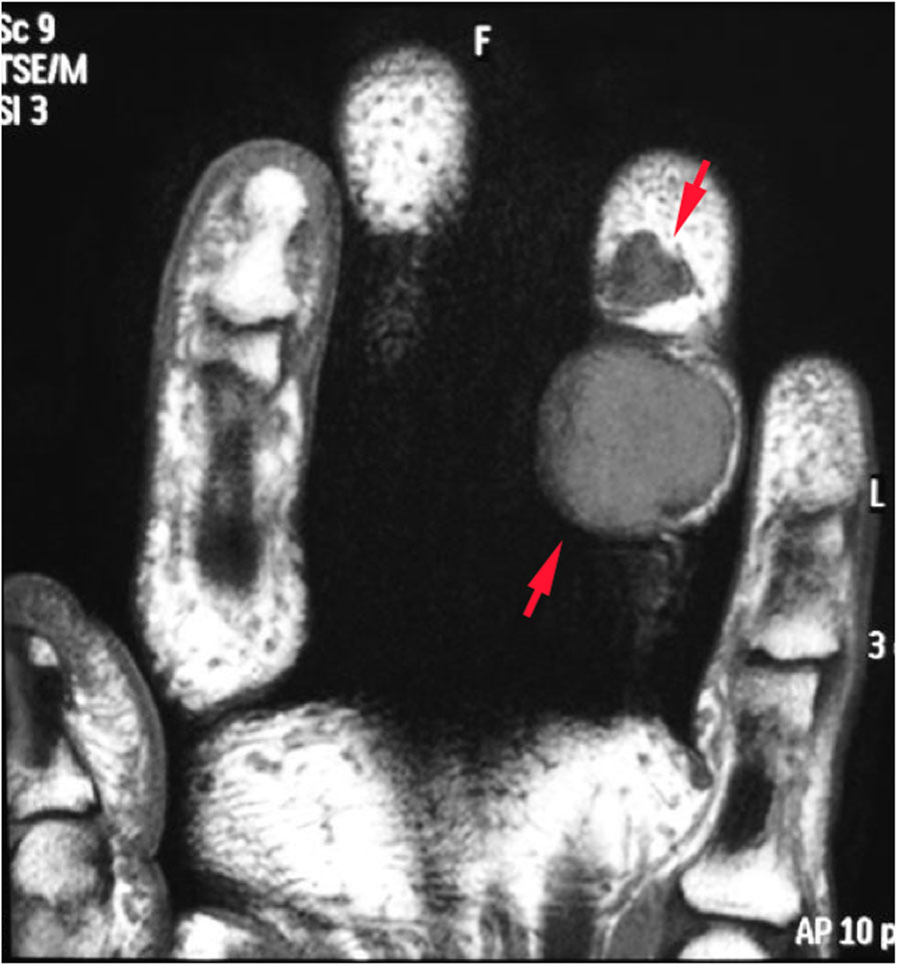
MRI can identify Al Qattan type 2 tumors where 2 or more distinct tumoral lobes exist
